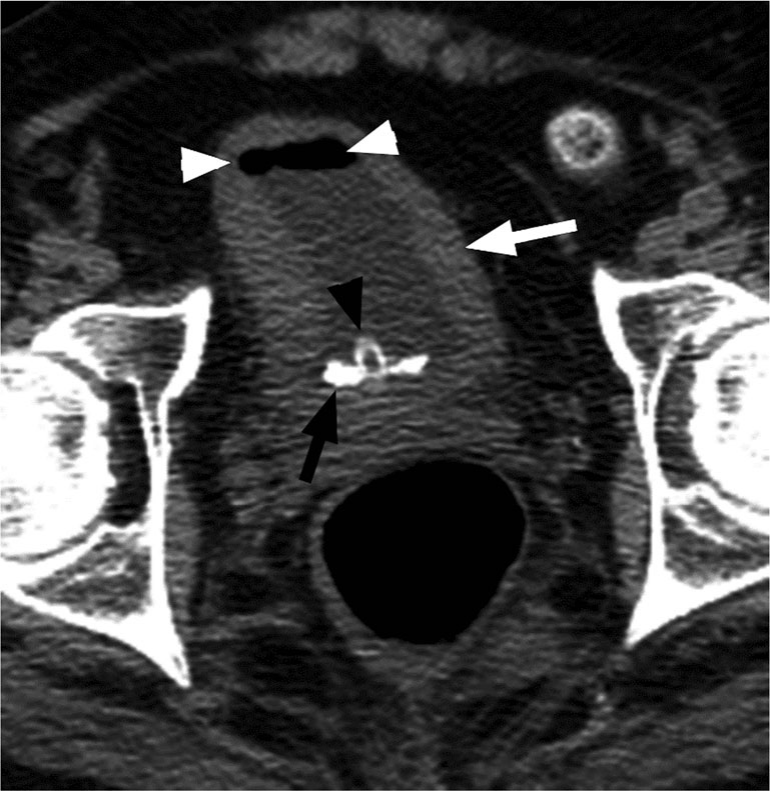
Neurogenic bladder with bladder stone formation. SCI patient with indwelling urinary catheter (*black arrowhead*). Non-contrast CT image shows circumferential urinary bladder wall thickening (*white arrow*) and a calcified bladder stone layering dependently (*black arrow*). Note catheter tubing within the bladder lumen (*black arrowhead*) causing gas in the urinary bladder (*white arrowheads*)
